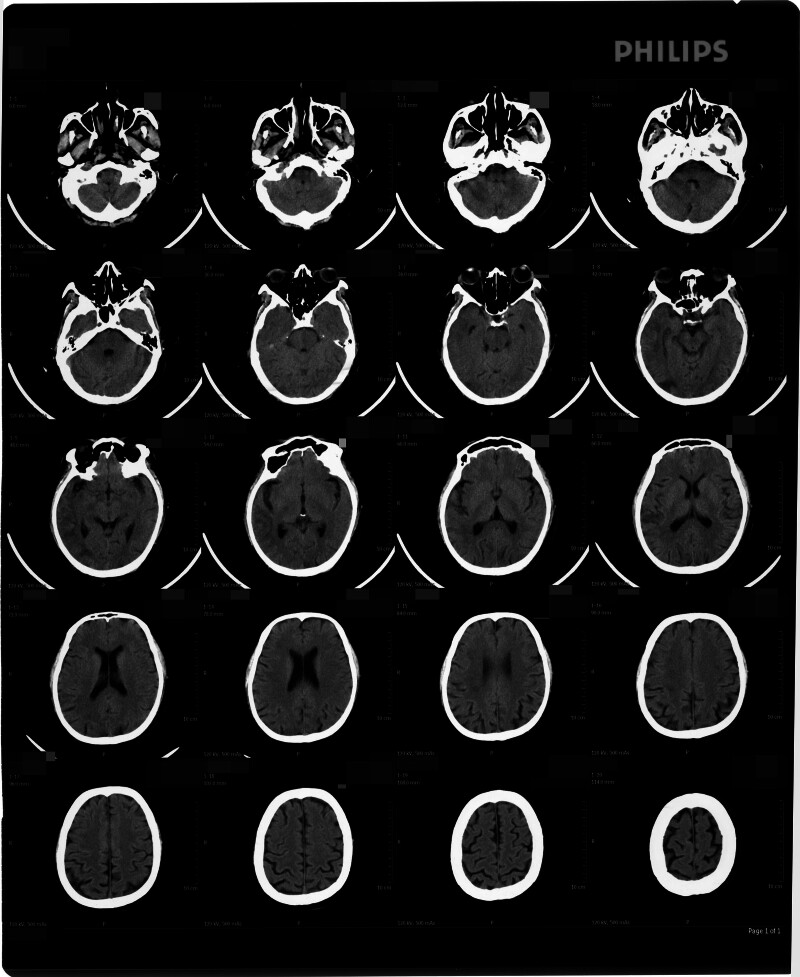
Cranial computed tomography imaging on May 27 showed improvement of the cerebral infarction compared with the results from May 13 and May 22.
